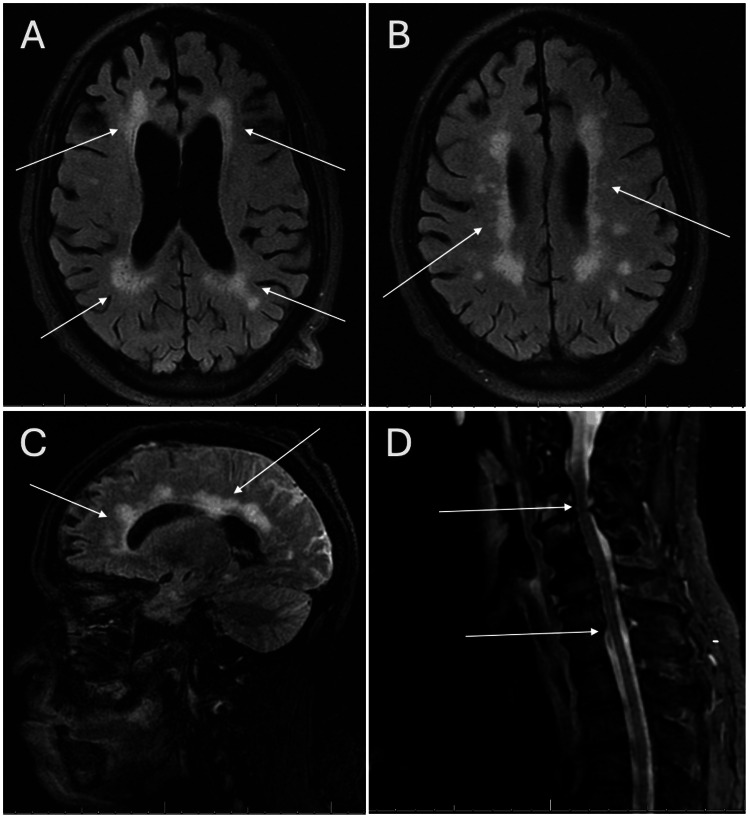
Brain MRI scan with and without contrast (A-C) showing non-enhancing confluent bilateral periventricular and deep white matter FLAIR hyperintensities likely related to sequelae of chronic ischemic small vessel disease, in addition to mild global cerebral volume loss with sulcal widening and ex vacuo dilation of the ventricles. These lesions were not enhanced. Cervical spine (D) MRI with and without contrast shows multilevel spondylitis changes without abnormal cervical cord signal alteration or post-contrast enhancement. MRI: magnetic resonance imaging; FLAIR: fluid-attenuated inversion recovery.
